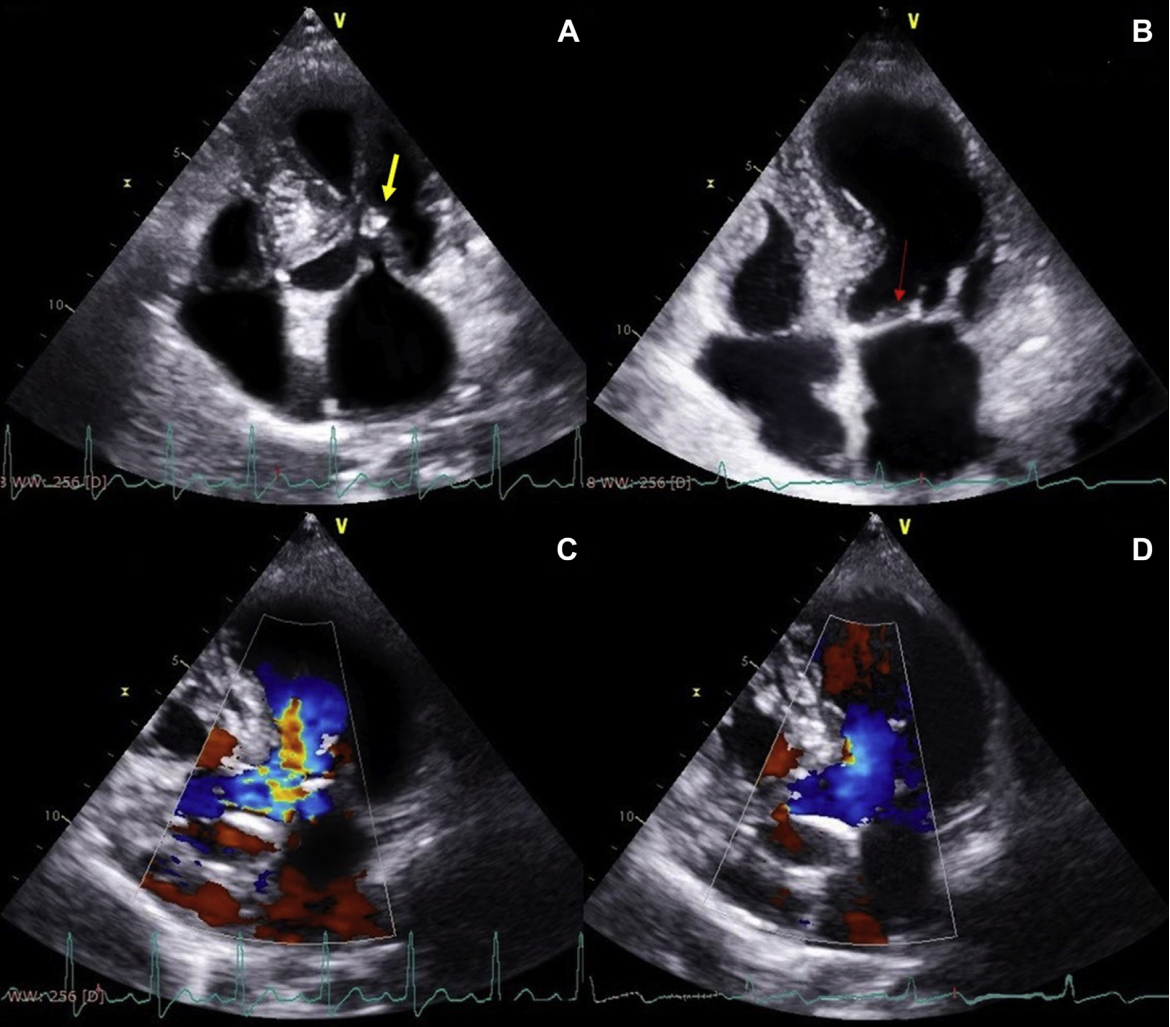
(A) Two-dimensional TTE apical four-chamber view showing systolic anterior motion of mitral leaflet (*yellow arrow*) proximate to the basal septum. (B) Two-dimensional TTE apical four-chamber view showing resolution of systolic anterior motion of mitral leaflet (*red arrow*) after revascularization. (C) Two-dimensional TTE apical five-chamber view with color Doppler evaluation showing turbulence at the LVOT due to dynamic outflow tract obstruction. (D) Two-dimensional TTE apical five-chamber view with color Doppler evaluation at LVOT showing resolution of turbulence at LVOT following revascularization.
